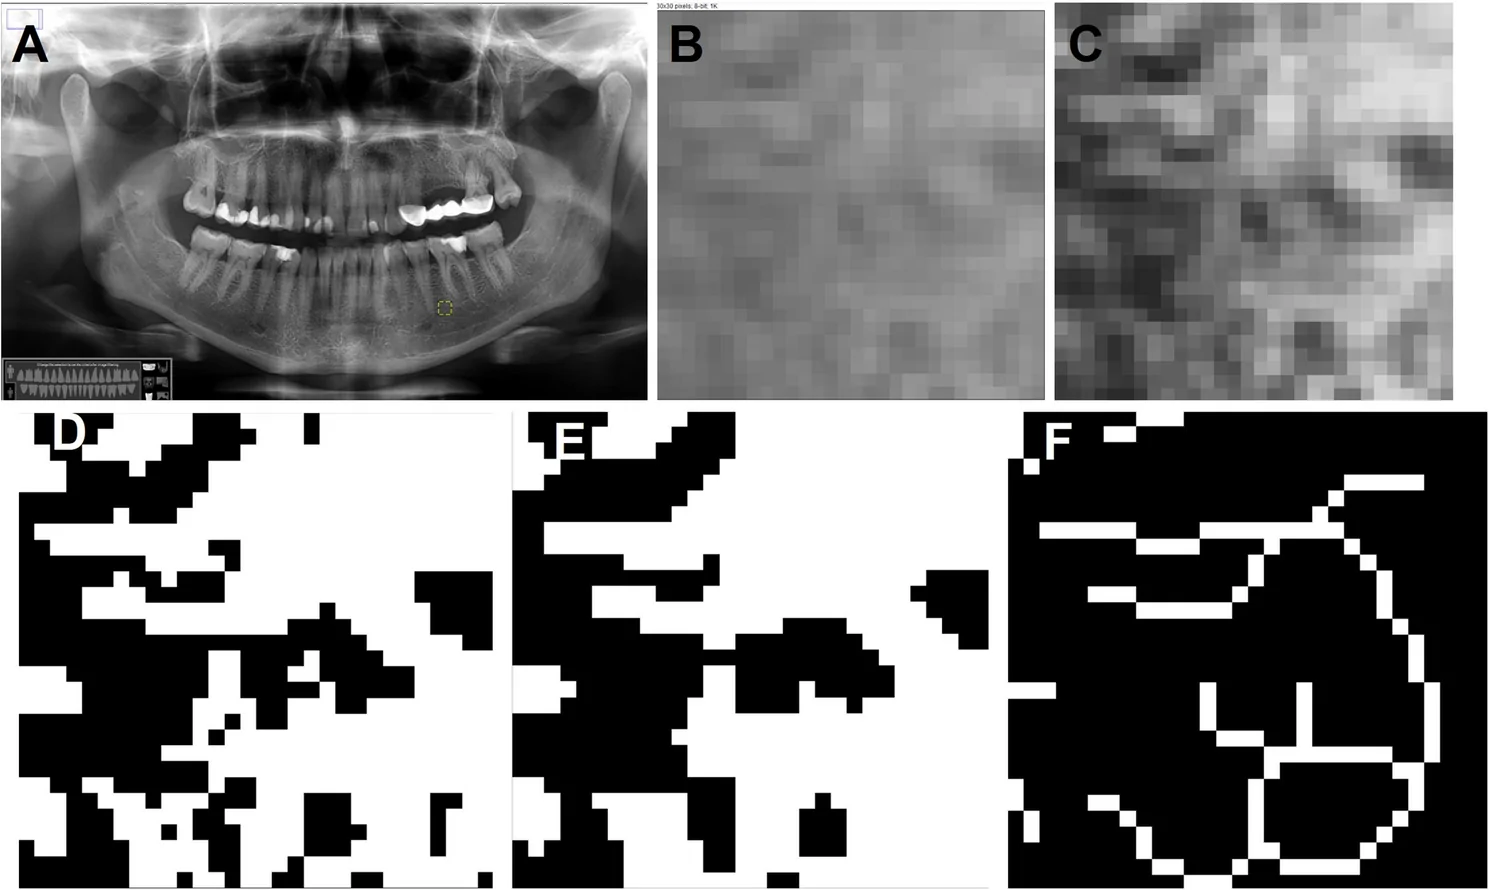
Sequential steps of the image processing protocol: (a) Panoramic radiograph with 30 × 30 pixel ROI, (b) 8-bit cropped ROI, (c) Contrast enhancement using CLAHE, (d) Binarization via Phansalkar thresholding, (e) Noise reduction with Despeckle, (f) Final skeletonized image for box-counting analysis
